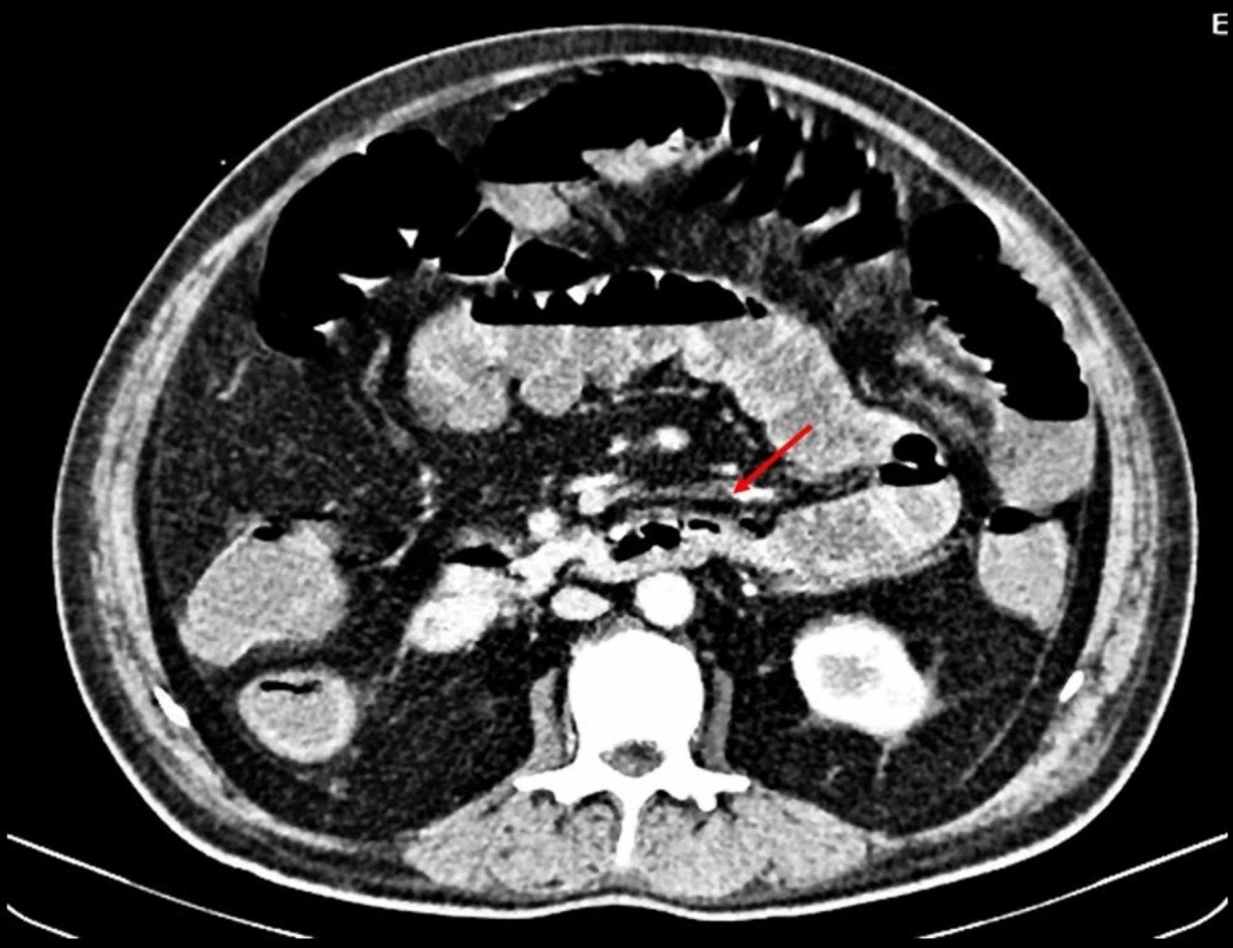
Superior mesenteric artery (SMA) thrombosis Contrast-enhanced CT of the abdomen showing an intraluminal filling defect in SMA indicating SMA thrombosis.
